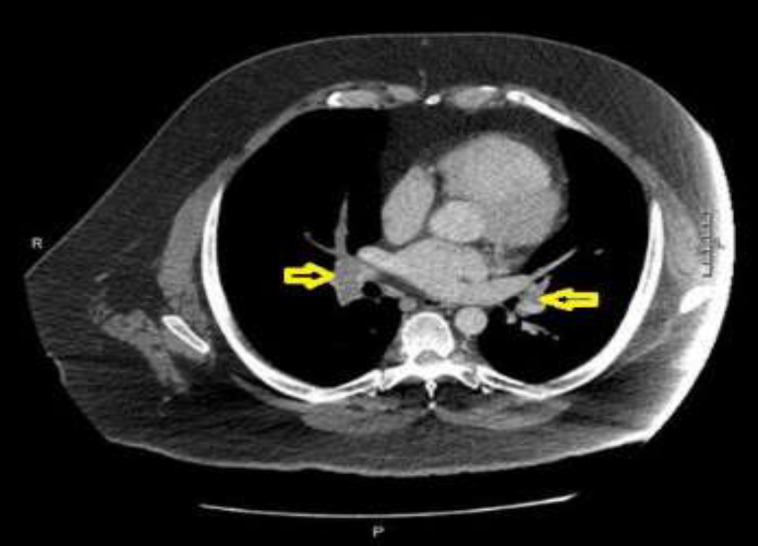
CT angiogram, axial view: Yellow arrows pointing at emboli (grey area) within pulmonary arteries
